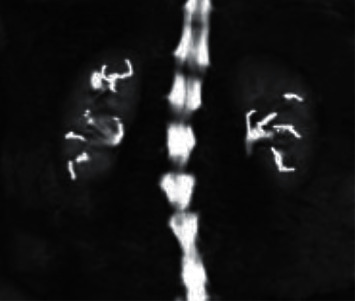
Ultrasound-guided image.
